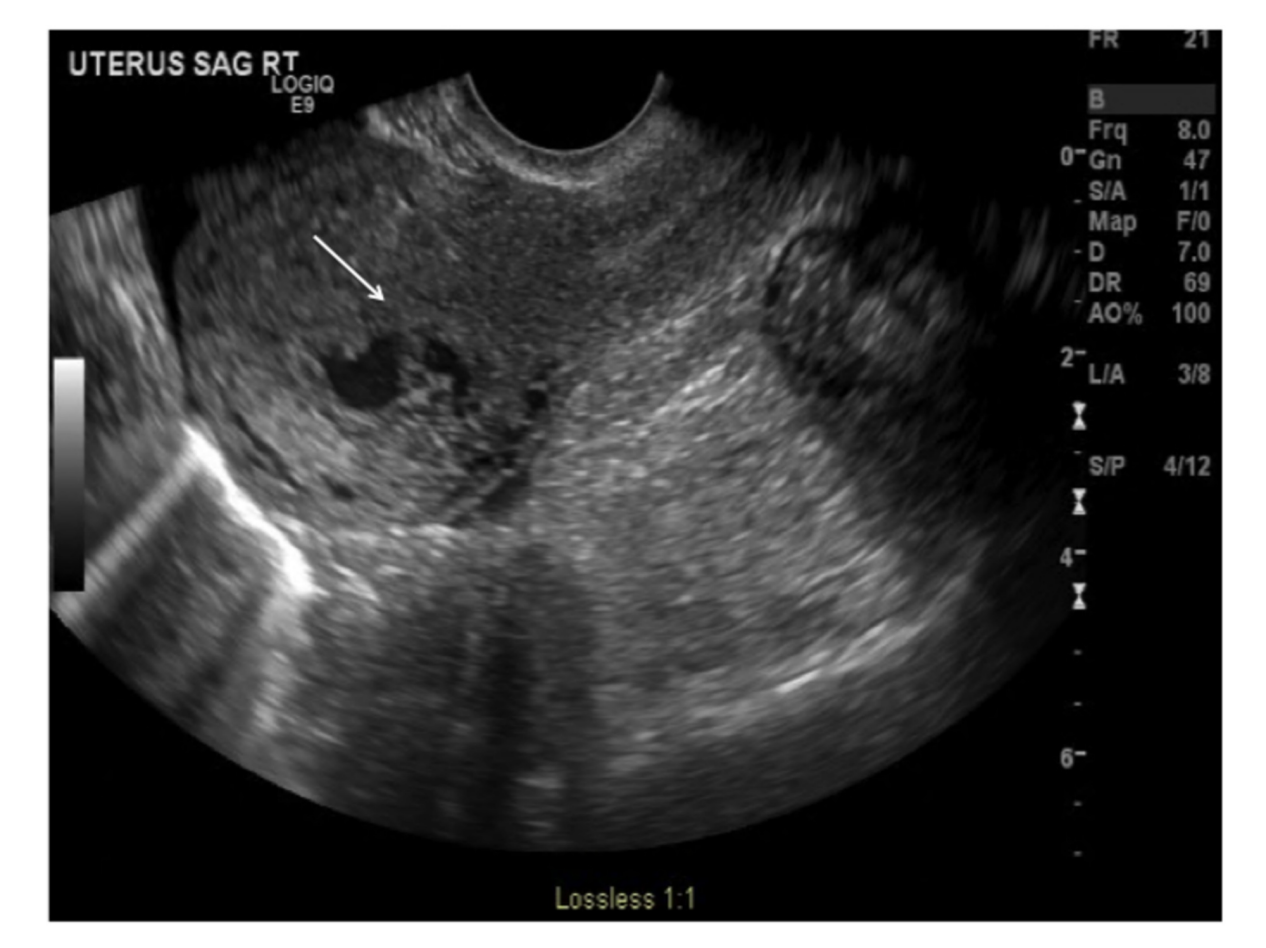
Transvaginal ultrasound of the uterus showing a hypoechoic lesion in the body of the uterus
